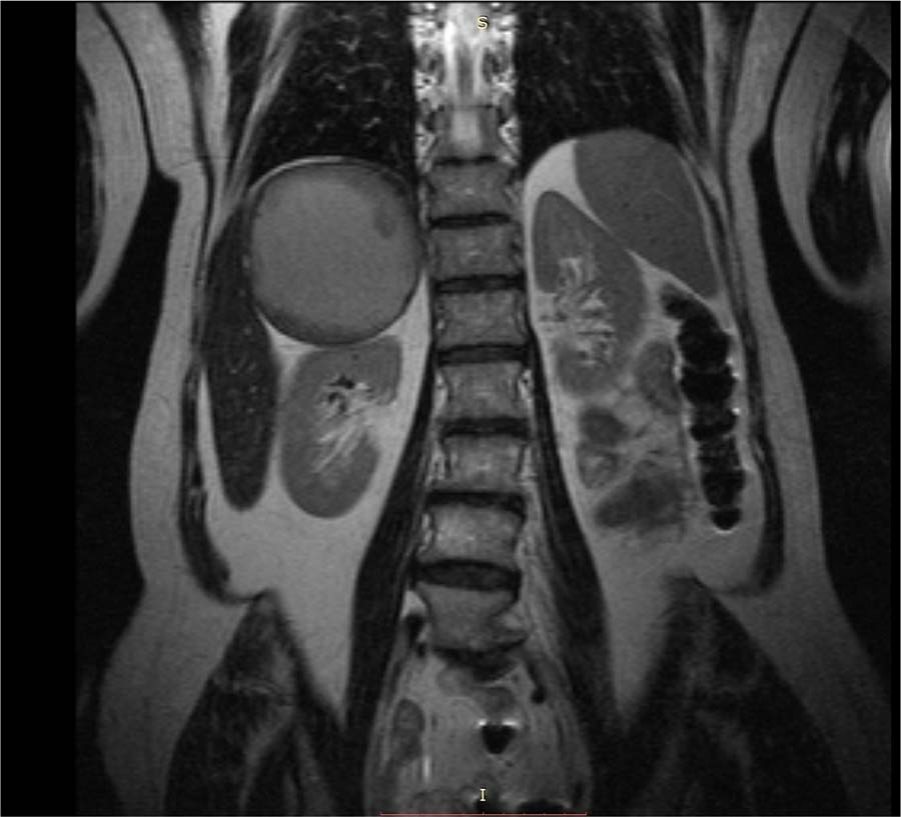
MRI caption showing a giant well-circumscribed right adrenal mass with solid components and thick fluid content. The remarkable dimension of the mass can be appreciated if compared to the right kidney.
